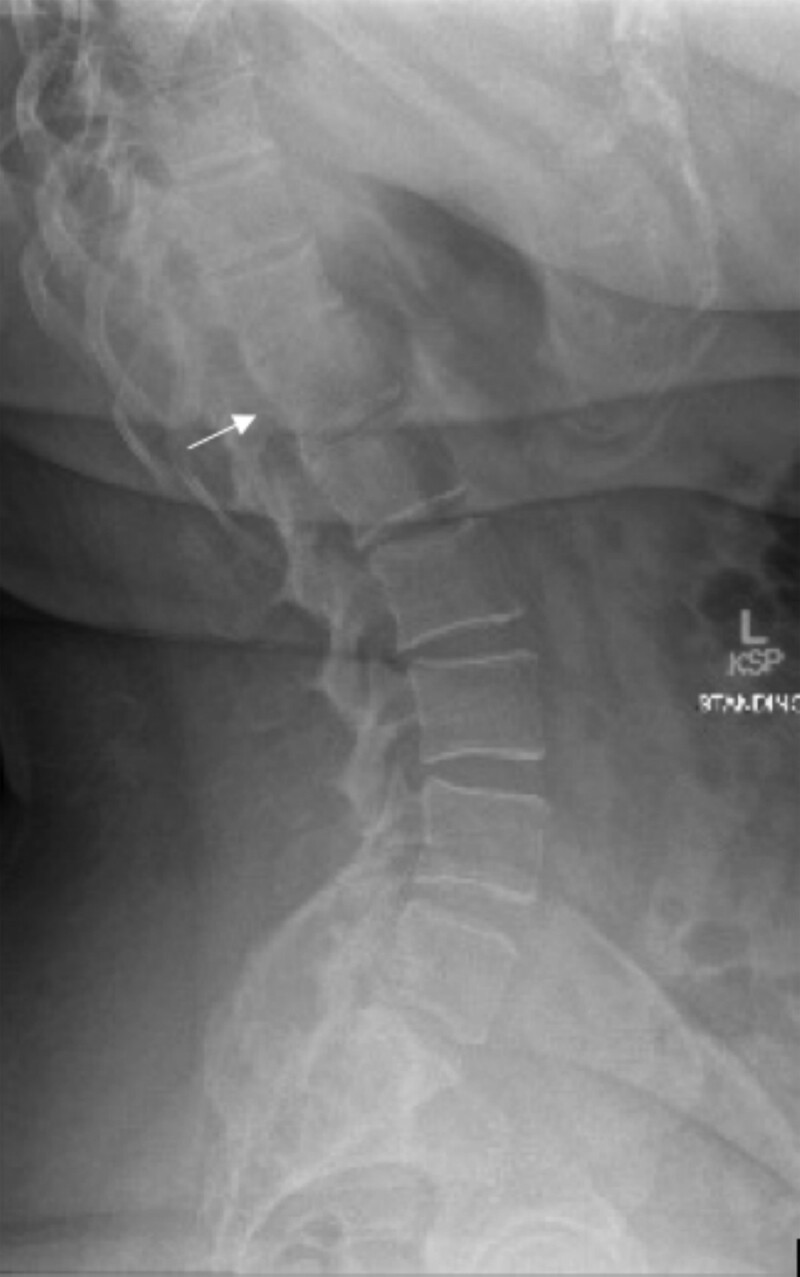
Lateral radiograph of the lumbar and thoracic spine revealed wedging of the T12 vertebrae (white arrow), consistent with a compression fracture of T12.
